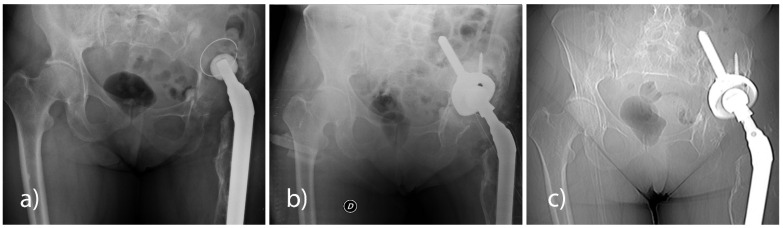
(a) Aseptic mobilization of the right hip prosthesis cup. (b) 6-month post-operative radiographic control shows the revised cup (Sansone cup with an iliac stem and RegenOss) stably in place. (c) A 12-month CT scan shows no signs of prosthetic loosening.
